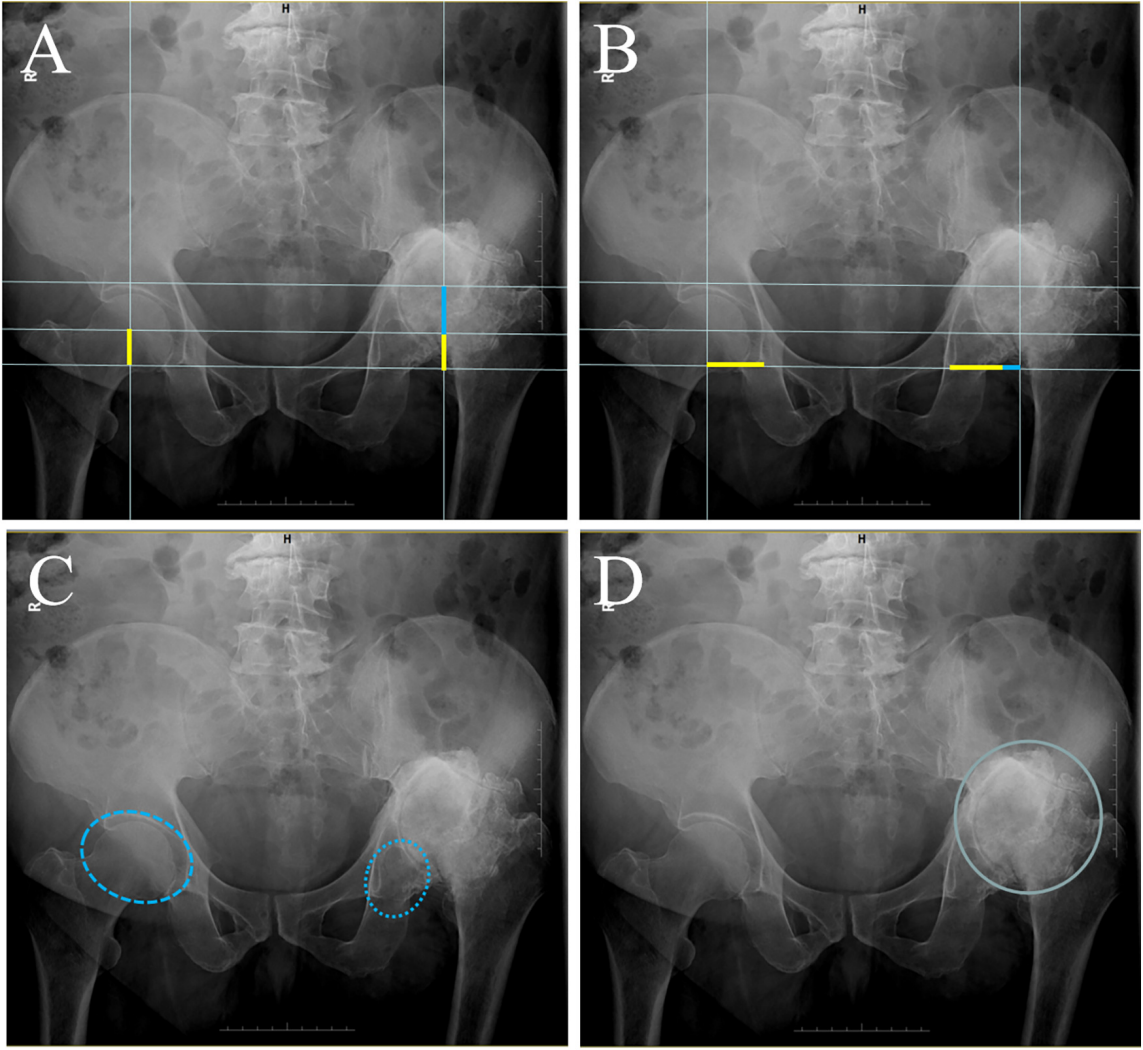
Anterior posterior view of X-ray radiological characteristics in patients with long-term unreduced hip joint dislocation. (A) The anterior posterior pelvic radiograph of the patient was placed on a standard grid line, and the vertical distance of the femoral head rotational center to the ipsilateral teardrop was measured bilaterally. The yellow lines indicate the distance of the femoral head rotational center on the healthy side to the ipsilateral tear drop in the vertical direction, and the blue lines indicate the portion of the femoral head rotational center on the affected side to the vertical distance to the teardrop on the ipsilateral side beyond the healthy side. (B) The anteroposterior pelvic radiograph of the patient was placed on a standard grid line, and the horizontal distance of the femoral head rotational center to the ipsilateral teardrop was measured bilaterally. The yellow lines indicate the distance of the femoral head rotational center on the healthy side from the ipsilateral tear drop in the horizontal direction, and the blue lines indicate the portion of the femoral head rotational center on the affected side from the horizontal distance from the teardrop on the ipsilateral side relative to the healthy side beyond. (C) In patients with long-term unreduced posterior hip dislocation, the femoral head on the affected side detaches from the original acetabulum, and a hypodense ovoid area, known as the image of the real acetabulum, can be seen in the position of the original acetabulum. (D) Quasi-circular high-density area can be found in the anteroposterior X-ray examination, similar to the lunar aureole (pseudoacetabulum) around the moon (femoral head) wrapped around the femoral head, and is called the “aureole sign.”
